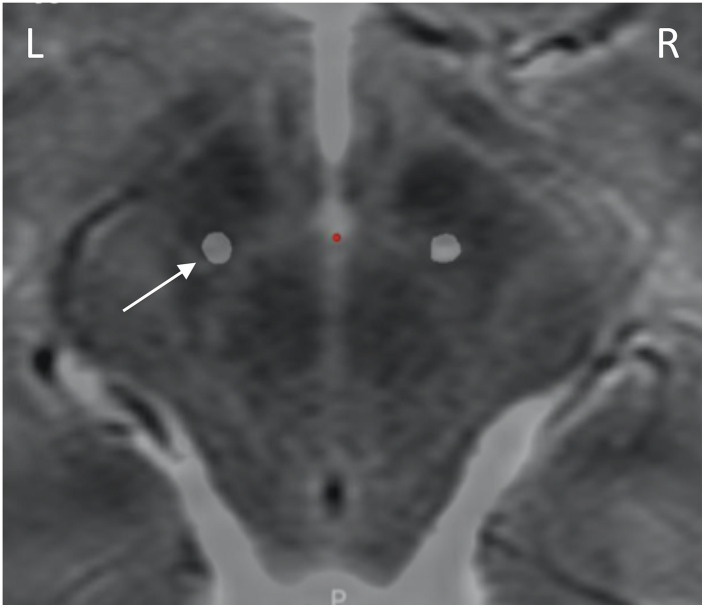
Lead placement. Preoperative axial T2 MRI at the level of the dorsal STN (4 mm inferior to the intercommissural line), with the lead locations identified by merging the postoperative CT scan via surgical planning software. Leads are within dorsolateral STN. Only the left lead (white arrow) was attached to sensing devices.
